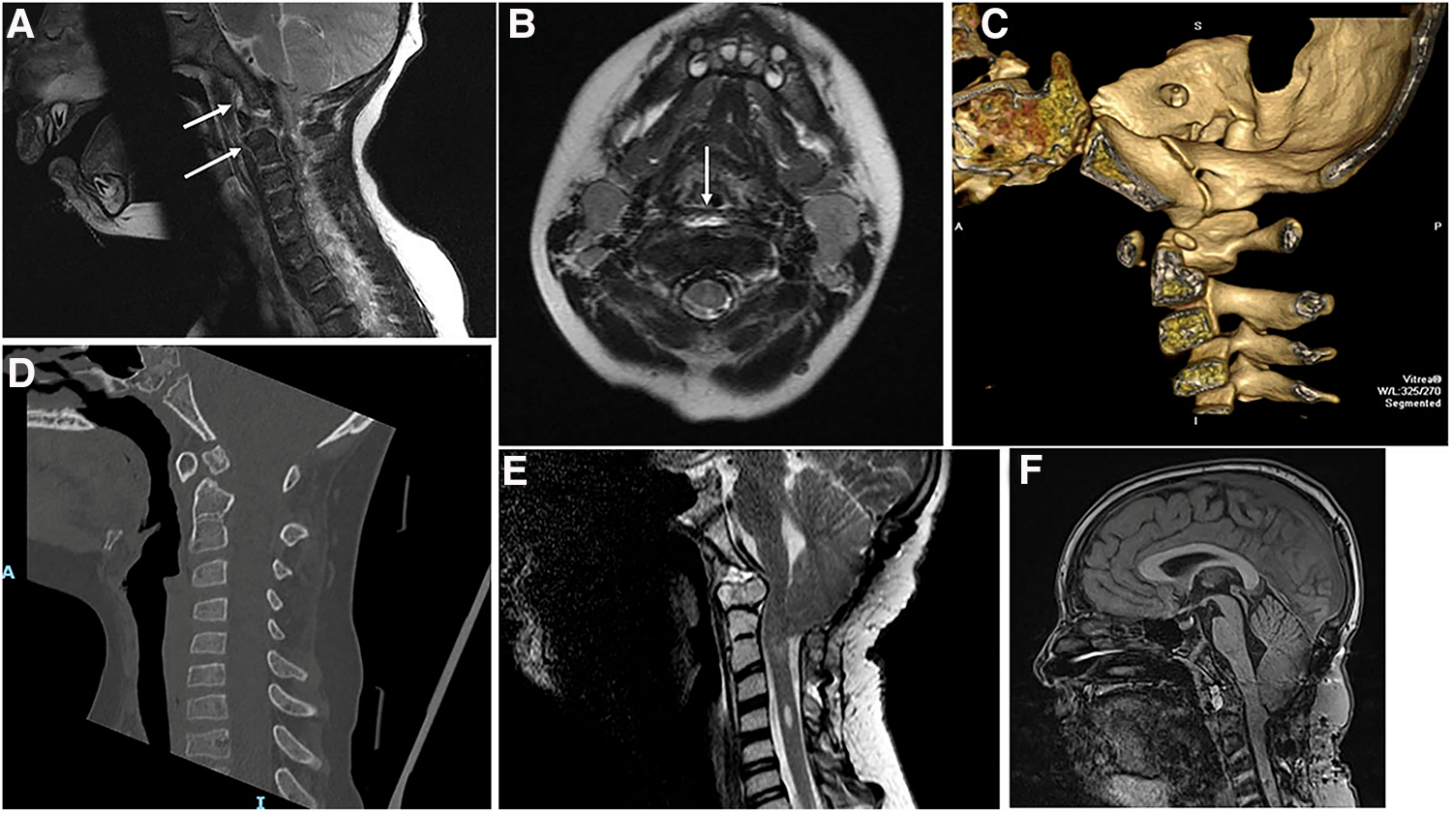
(A) Midsagittal T2-W MRI of CVJ of an 18-month-old boy. There is a prevertebral bright signal in front of C1–C2 vertebral bodies, (arrows) Chiari I abnormality, and a widened cervicomedullary junction. (B) Axial T2-W MRI through C1of the same patient as in (A). Note the prevertebral signal abnormality (arrow). (C) 3D CT of CVJ in midsagittal reconstruction made at the age of 2.5 years. A small ossicle is seen above the odontoid. (D) 2D CT of CVJ reveals an os odontoideum at the age of 10 years. (E) Midsagittal T2-W MRI of CVJ after posterior fossa decompression and dorsal occipitocervical fusion. There is ventral compression by the os odontoideum. (F) Midsagittal T2-W MRI of CVJ done after transoral resection of the os odontoideum.
